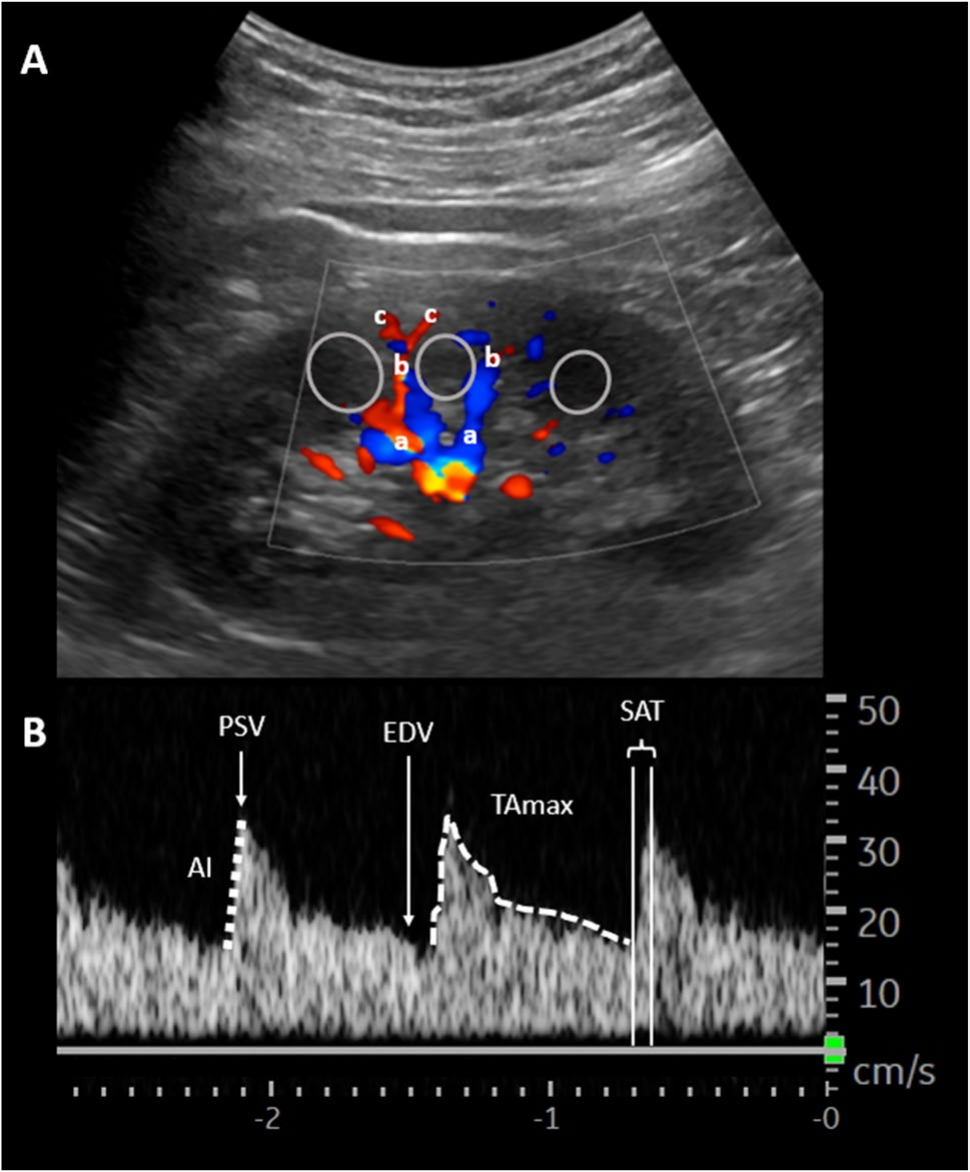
A Long axis view of Doppler renal ultrasound and B spectral wave form of interlobar artery. Pyramids/medulla (grey circles), segmental vessels (a), interlobar vessels (b) and arcuate vessels (c). Acceleration index (AI), peak systolic velocity (PSV), end-diastolic velocity (EDV), time-averaged maximum velocity (TAmax), and systolic acceleration time (SAT)
